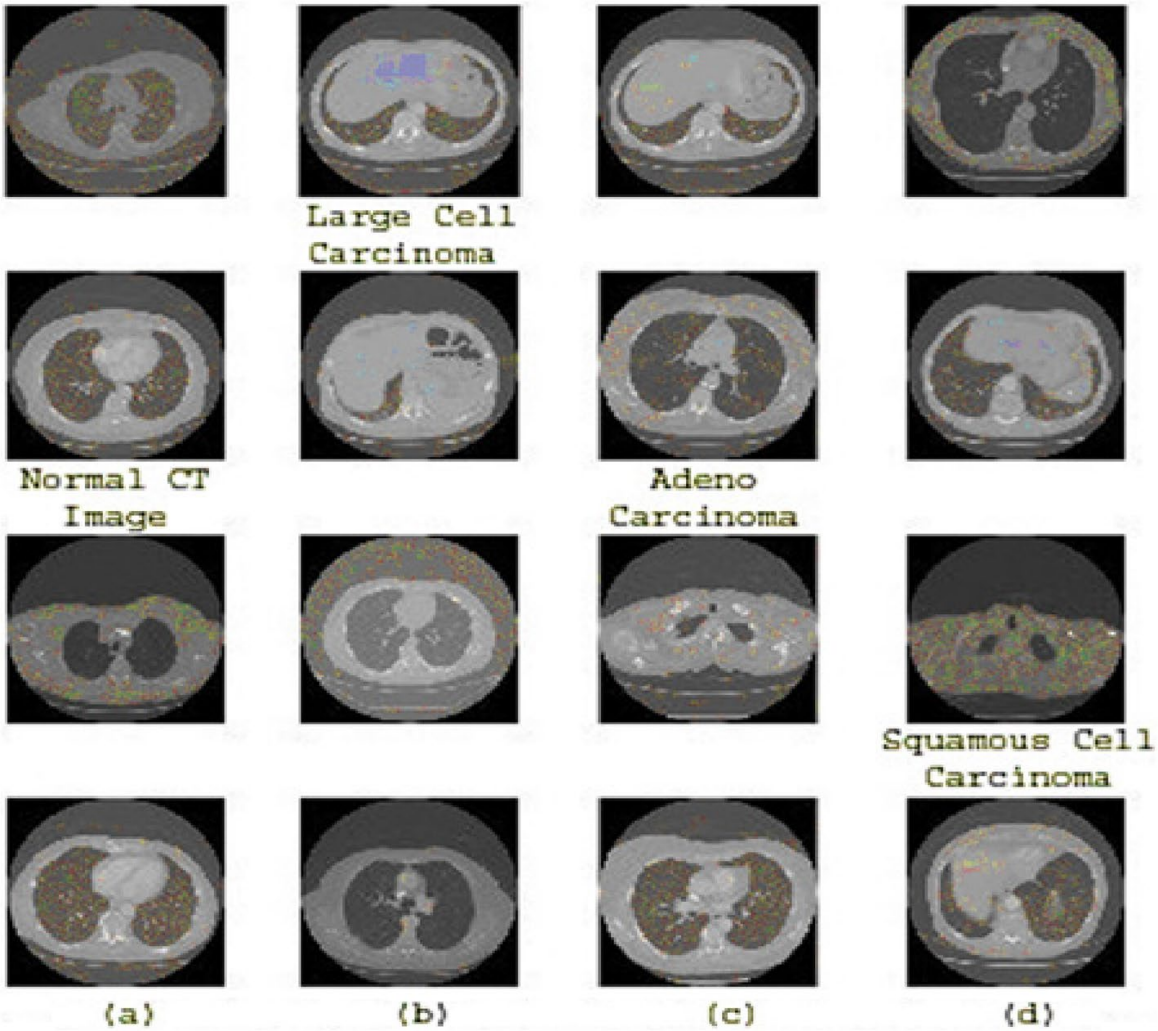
(a) Normal CT Image, b Large Cell Carcinoma, c Adenocarcinoma, d Squamous Cell Carcinoma
